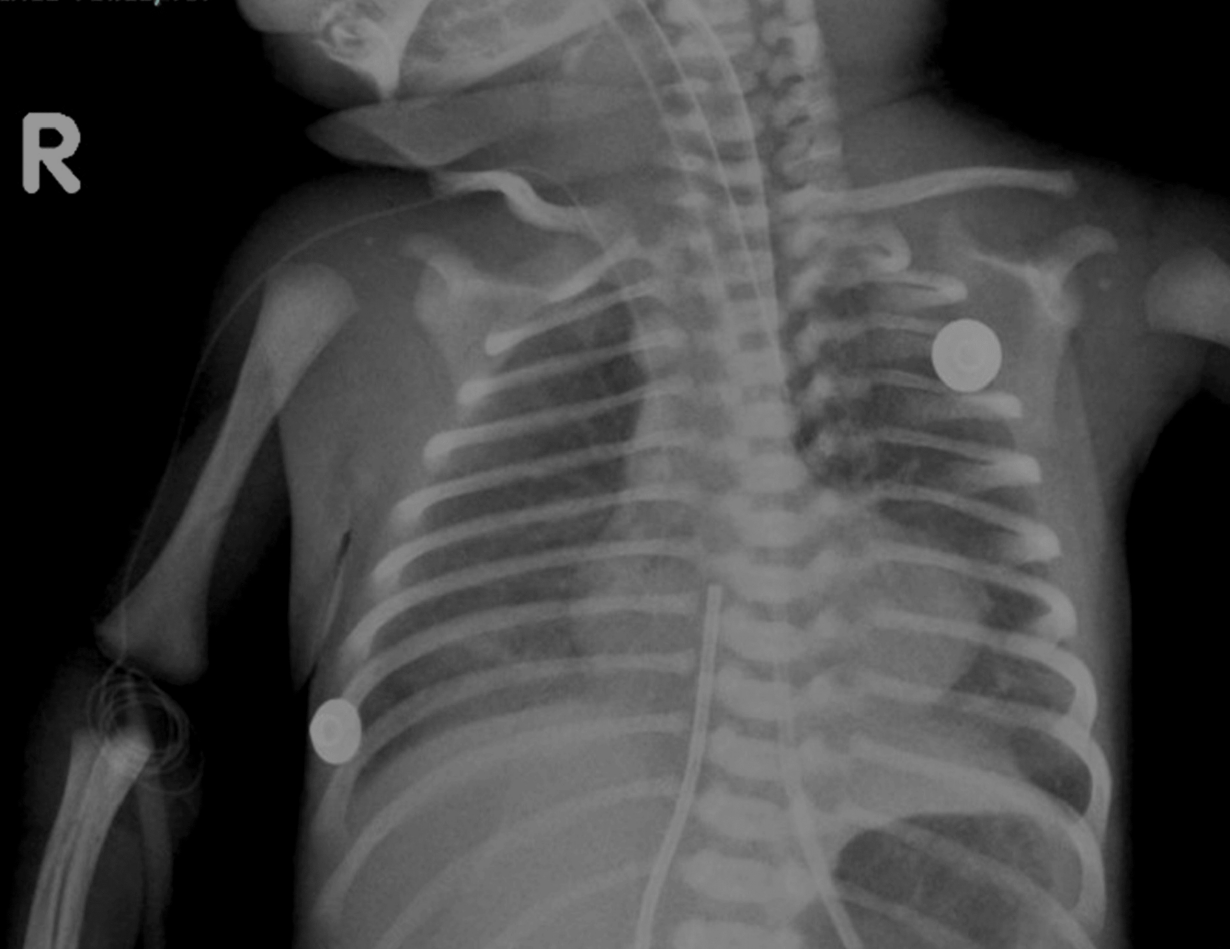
Chest radiograph. The initial radiograph shows elevation of the cardiac apex, a boot-shaped heart sign, and interstitial infiltrates in both lungs.
